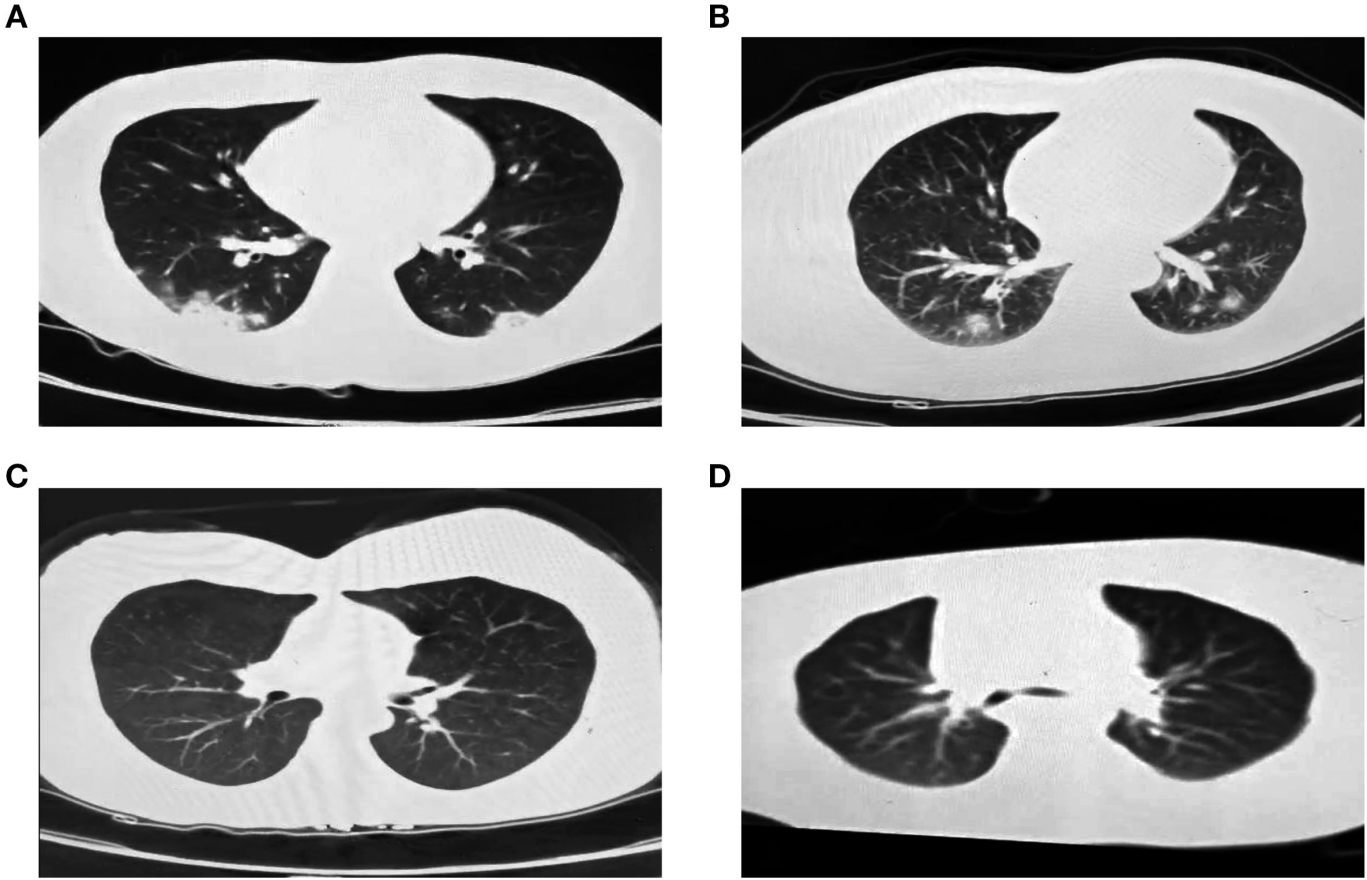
Chest CT images of the four family-clustered cases. (A) Case 1. (B) Case 2. (C) Case 3. (D) Case 4.
